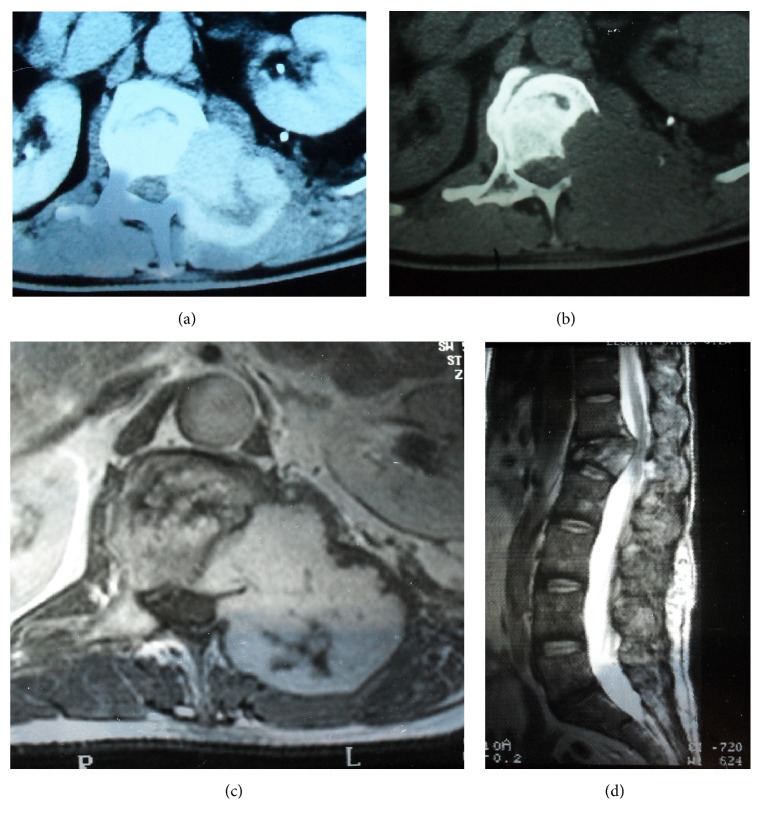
(a) CT: enhancing mass that has destroyed the left half of the body, left pedicle, and left transverse process of the L1 vertebra. (b) CT: bone window, partial destruction of the L1 body, absence of the left pedicle, and left transverse process. (c) MRI, axial T_1_WI, with gadolinium: mass of L1 vertebra destroying the body and compressing the paraspinal soft tissue. (d) MRI, sagittal T_2_WI: the hemangioma invading the spinal canal.
